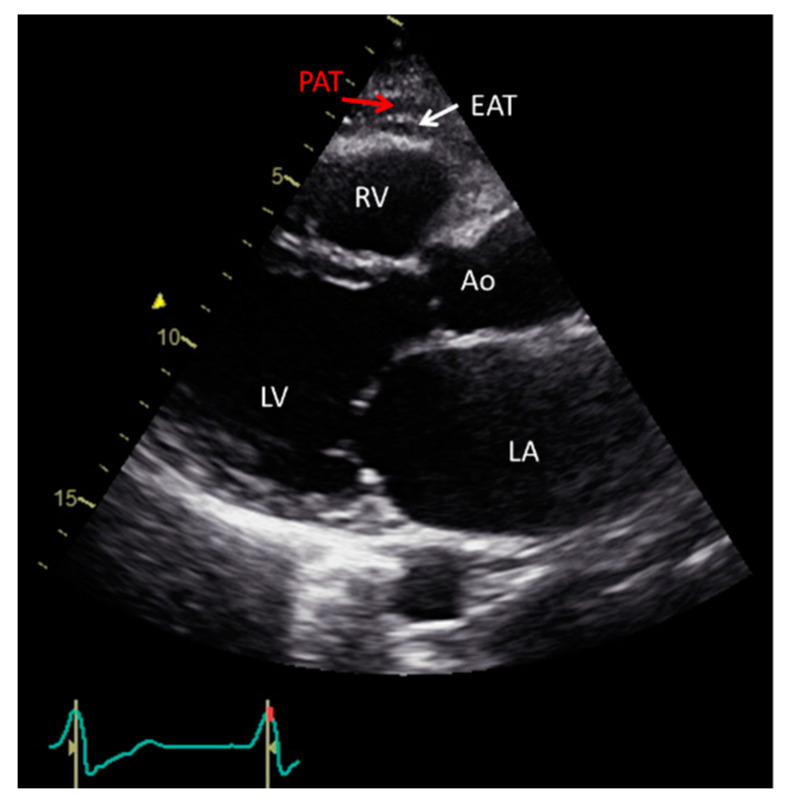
Transthoracic echocardiographic view showing EAT and PAT as echo-lucent areas in front of the RV free wall. EAT is pointed by a white arrow and PAT by a red arrow. Ao: aorta; EAT: epicardial adipose tissue; LA: left atrium; LV: left ventricle; PAT: pericardial tissue; RV: right ventricle.
